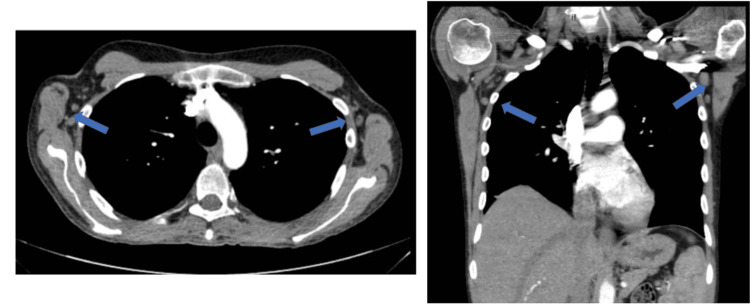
CTA of chest demonstrating bilateral axillary lymphadenopathy (blue arrows). CTA: Computed tomography angiography.
